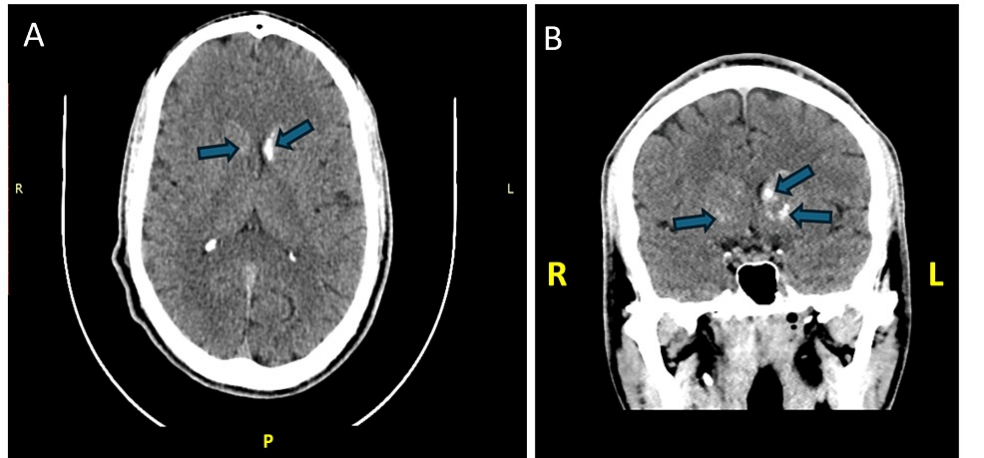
Brain CT scan images (A, axial section; B, coronal section) of case 2 showing bilateral calcifications in the caudate nucleus and lenticular nuclei (blue arrows). CT: computed tomography
